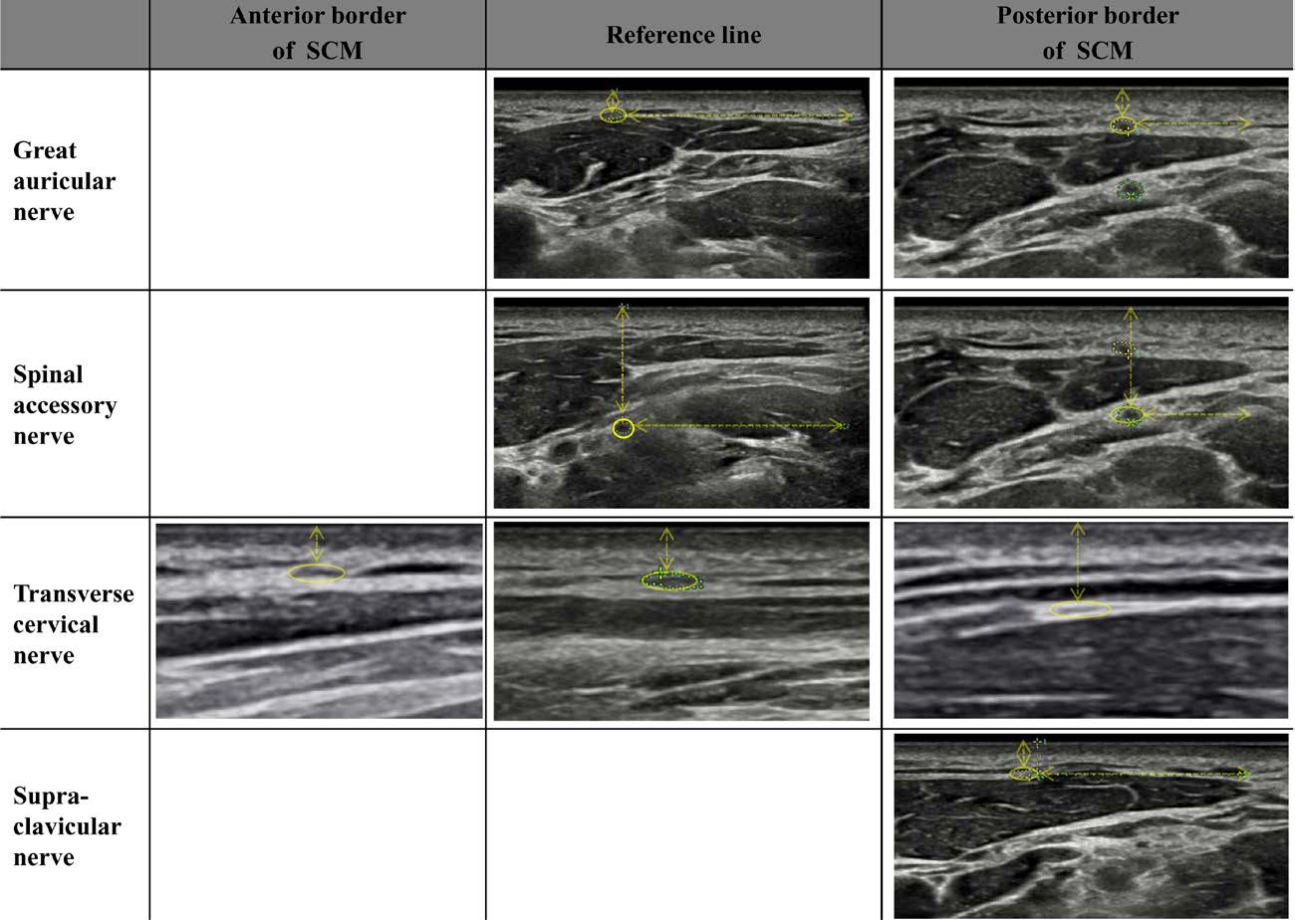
Ultrasonographic images of each nerve at the anterior border, reference line, and posterior border of SCM. Great auricular, spinal accessory, transverse cervical, and supraclavicular nerves were visualized with the transverse view of the path of each nerve. We measured cross-sectional areas, depth from the skin, and distance from the posterior border of the SCM. SCM = sternocleidomastoid.
